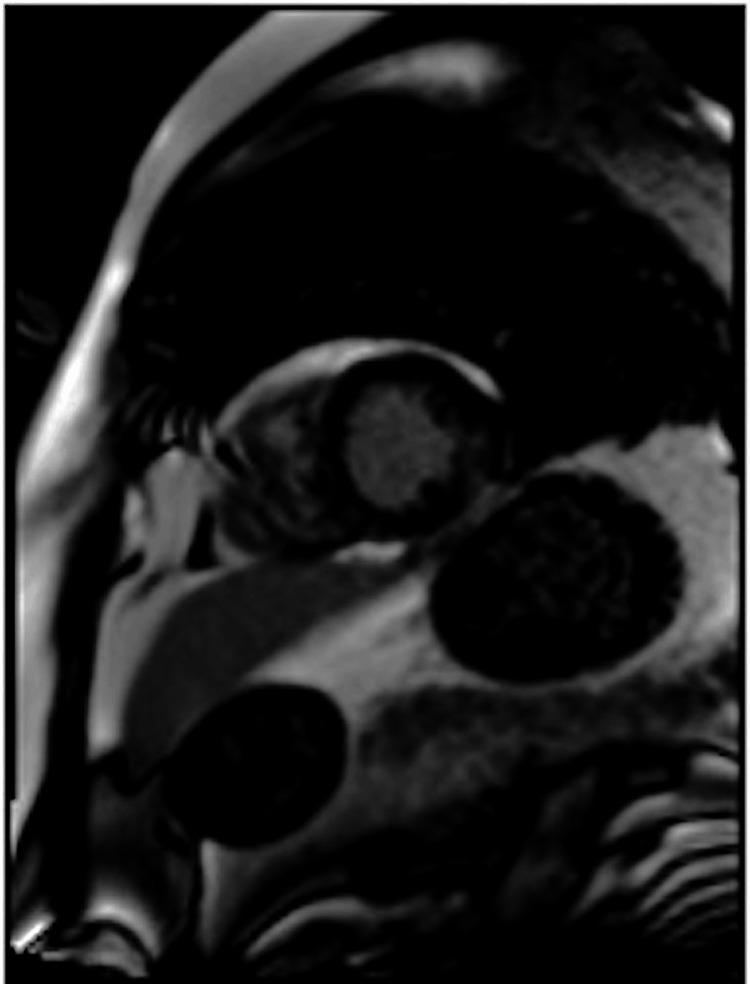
Follow-up MRI short axis view showing multifocal, patchy, mid-myocardial late gadolinium enhancement
